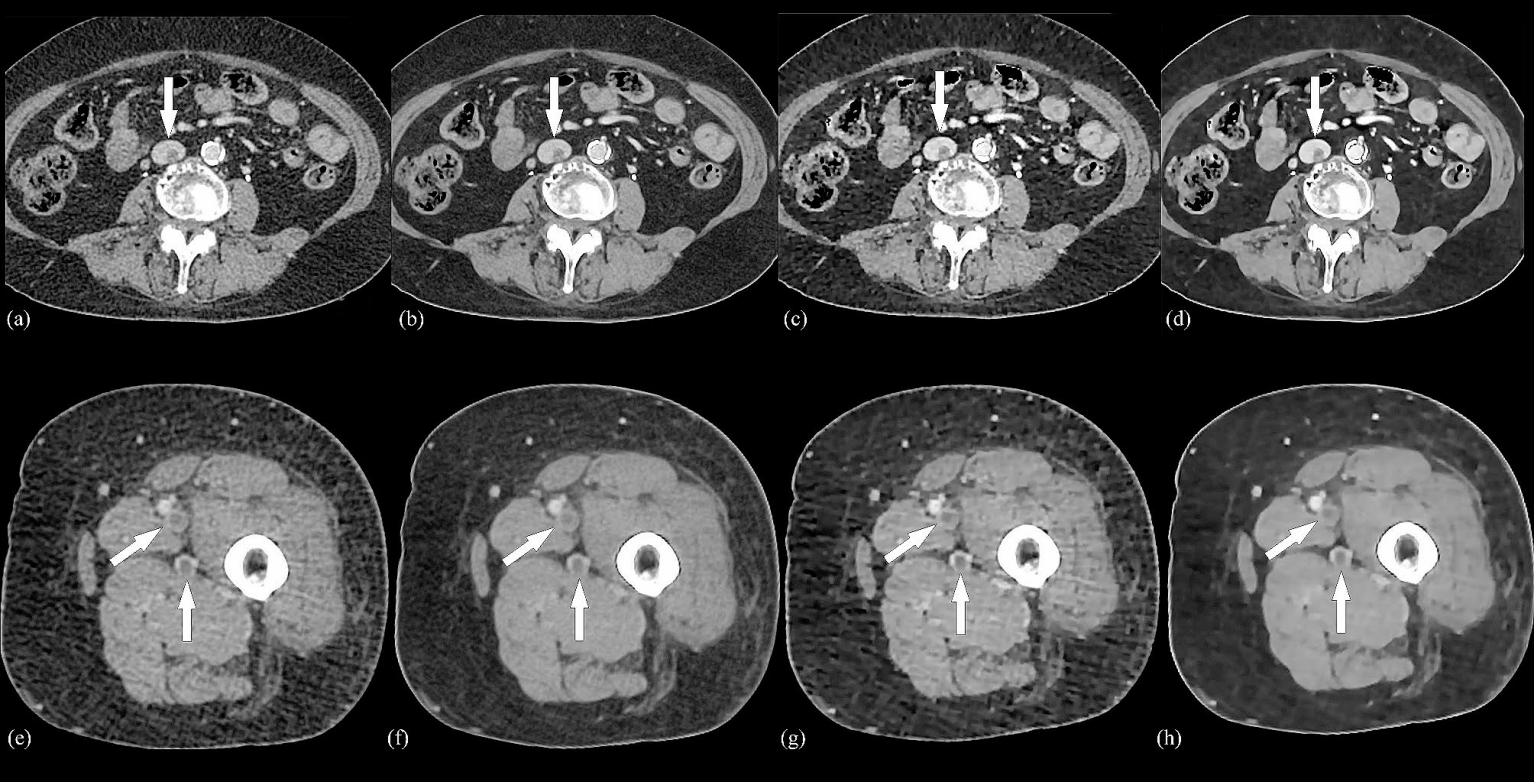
Axial CTV images of a 74-year-old patient with thrombosis in the inferior vena cava veins of the left lower extremity. AIDR 3D (a, e), AiCE (b, f), AIDR 3D-boost (c, g) and AiCE-boost (d, h). The clots within IVC (arrow, a-d), superficial femoral vein, and deep femoral vein in the upper left thigh(arrow, e-h)were well delineated and had a higher CT enhancement, SNR and CNR in AIDR 3D-boost, and AiCE-boost images than AIDR 3D and AiCE images. AiCE and AiCE-boost images showed a lower noise than AIDR 3D and AIRD 3D-boost images
